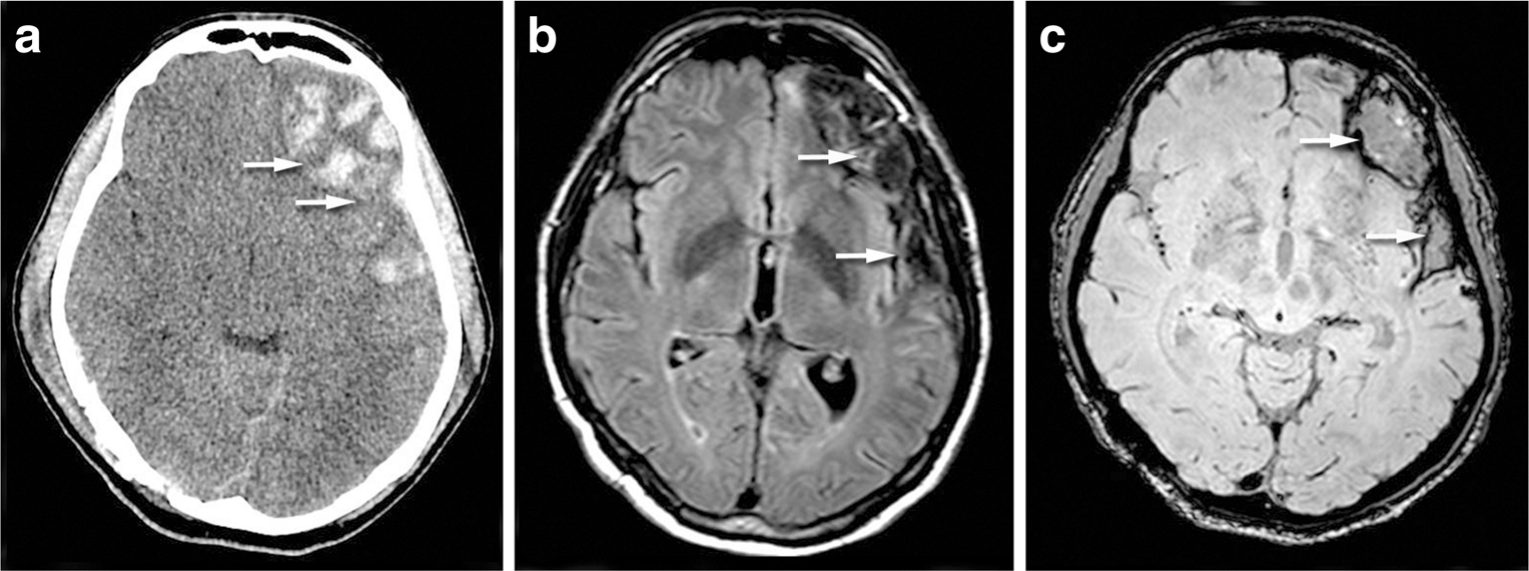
Brain haemorrhage in a patient with contralateral auditory agnosia. CT view (a) showing left fronto-temporal hemorrhagic injury. FLAIR sequence (b) and susceptibility-weighted imaging (c) showing the cortical damage in particular in the superior temporal gyrus and in the basifrontal region (*white arrows*)
